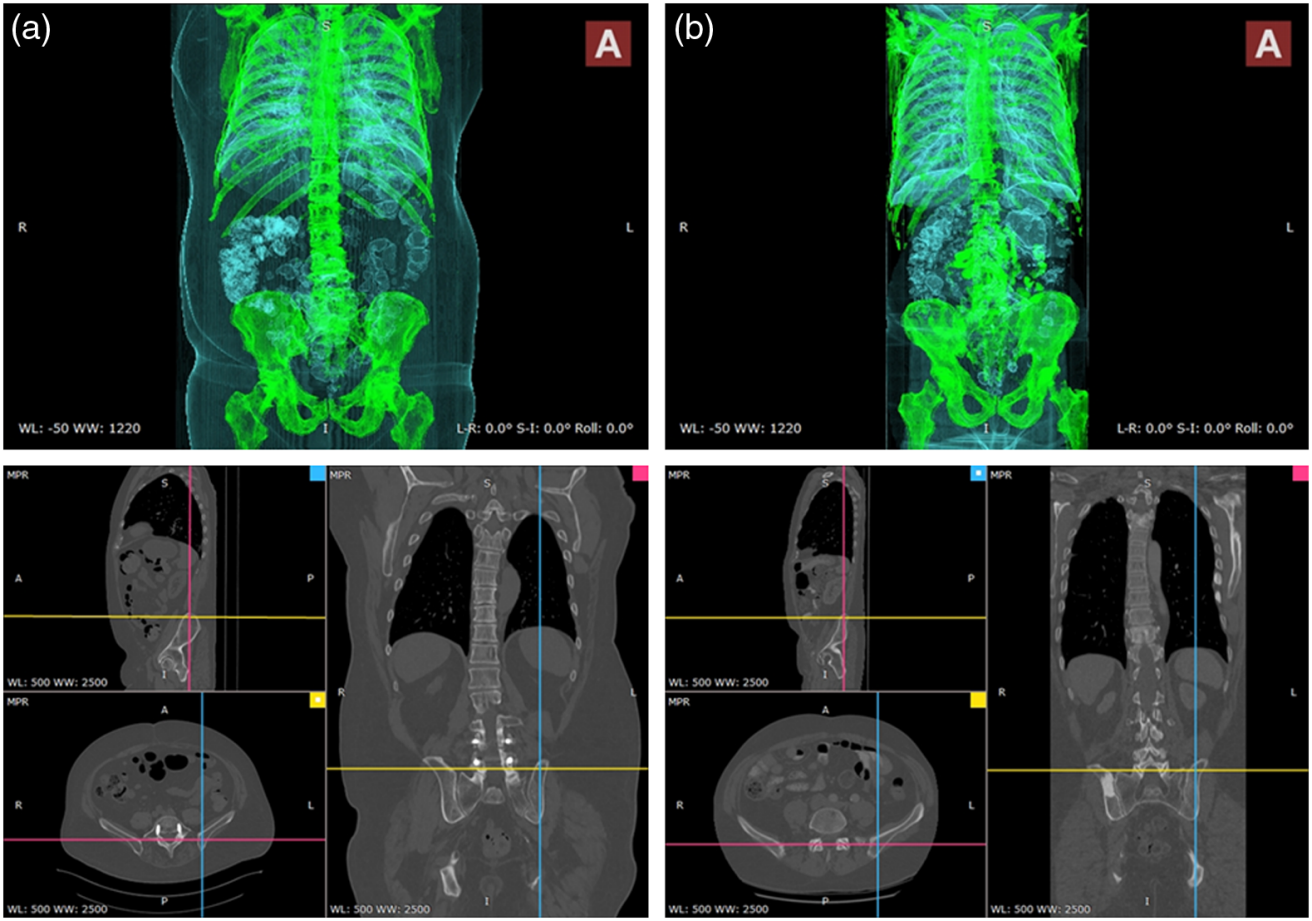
Registered field of view in CT data of patients A and B (Appendix A1).
